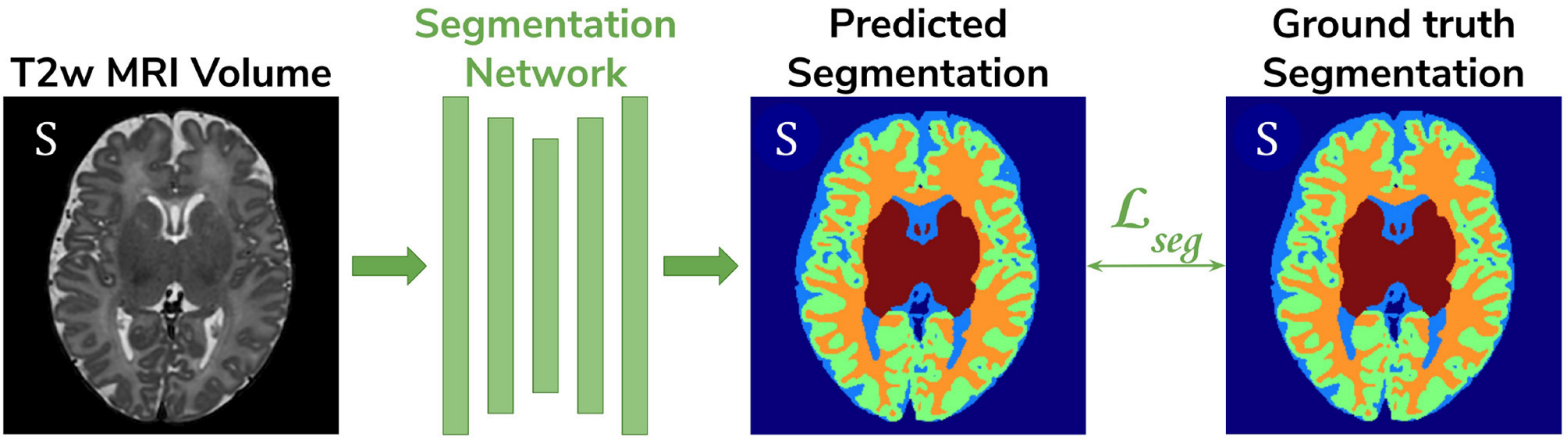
The baseline model consists of a 3D U-Net trained to segment source (dHCP) volumes. The input *T*2w MRI images, the predicted segmentation and the Draw-EM output segmentations are marked with S as they all belong to the source (dHCP) dataset.
